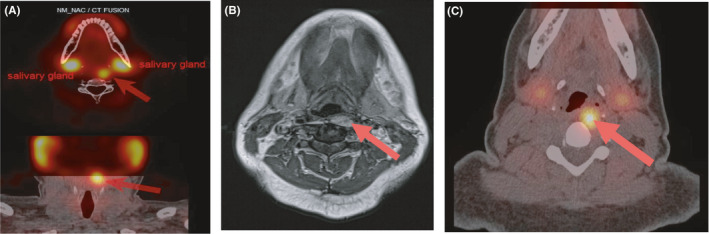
Images show a left retropharyngeal nodule, corresponds to a possible ectopic parathyroid lesion at: (A) ^99m^Tc sestamibi SPECT/CT, (B) MRI (T1 axial), and (C) 18F‐fluorocholine PET/CT respectively
